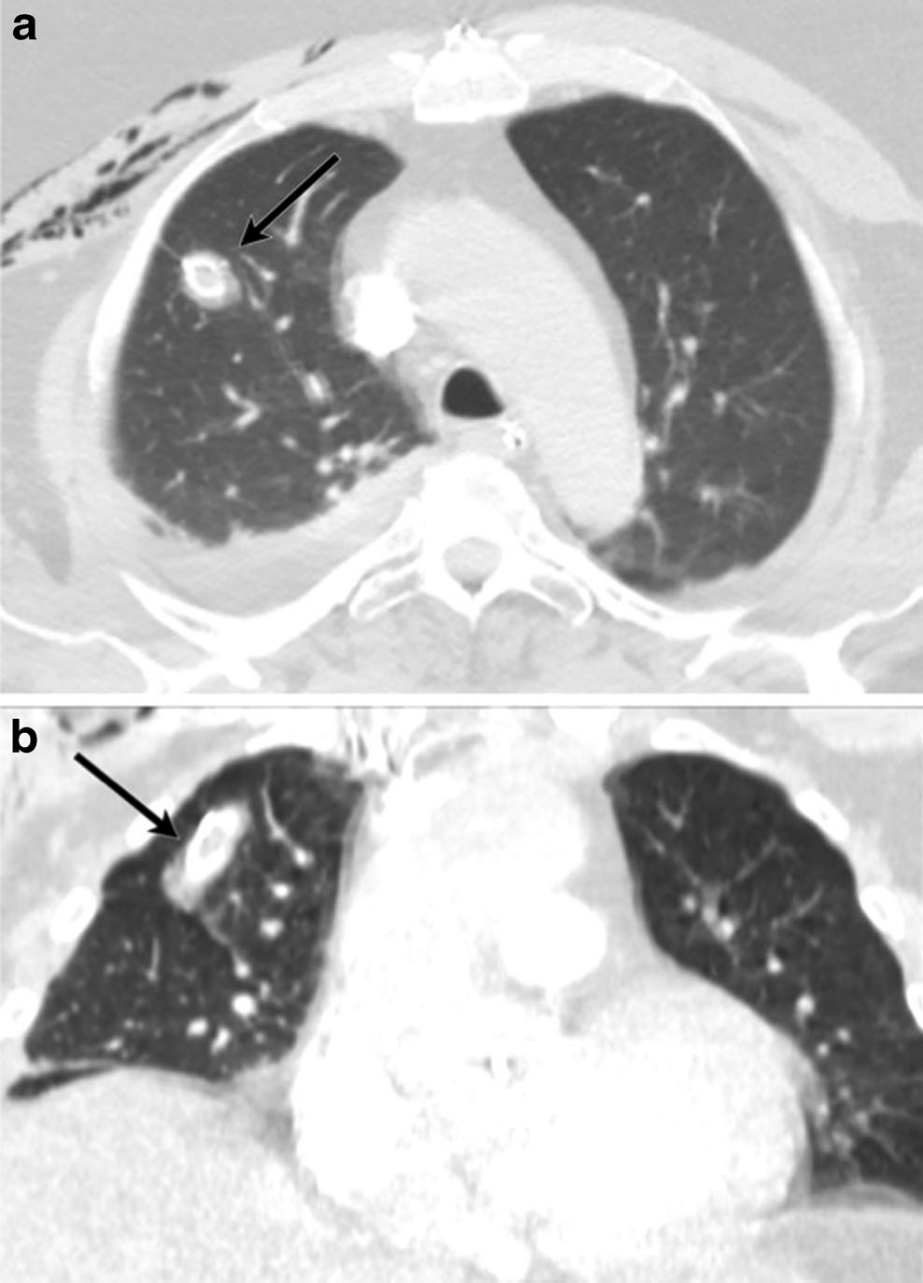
A 69-year-old male struck by a motor vehicle. CT was obtained to assess etiology of a non-resolving pneumothorax. Axial (a) and coronal (b) CT images through the chest demonstrate intra parenchymal placement of chest tube within the right upper lobe. Ground glass opacity (*arrow*) surrounding the chest drain represents lung laceration. Incidentally noted right pleural fluid and right anterior chest wall soft tissue emphysema
